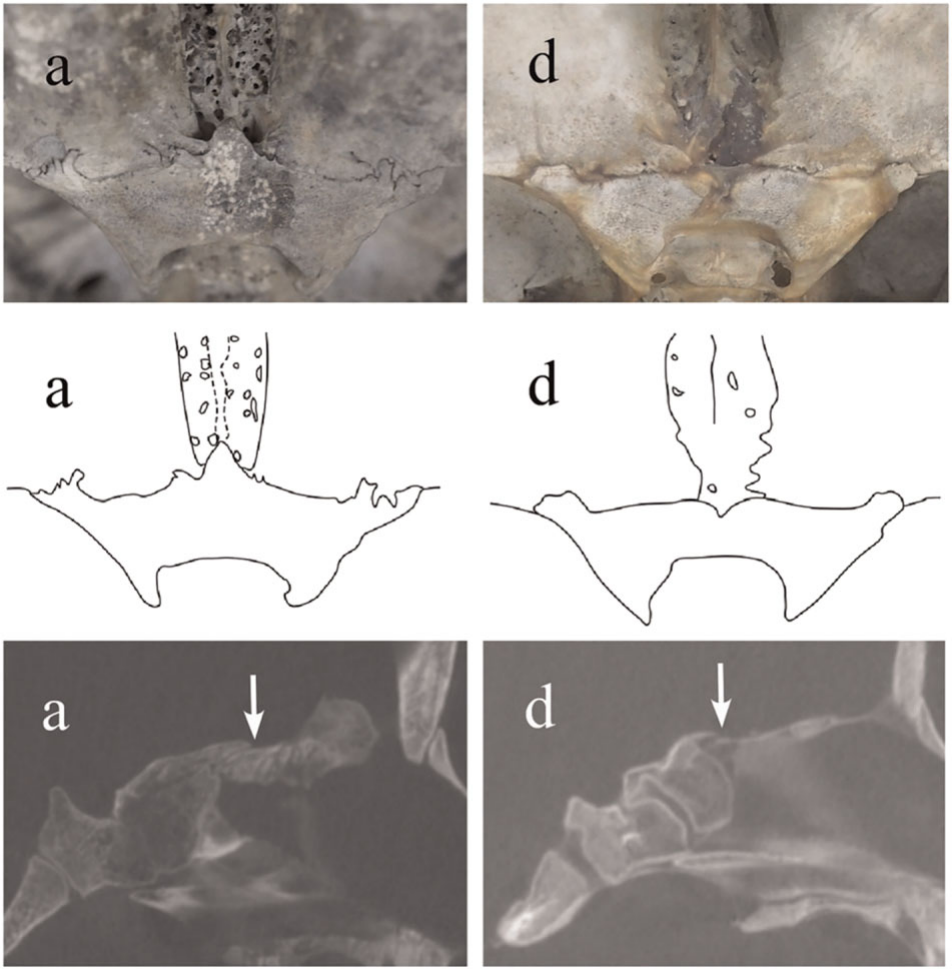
Morphological variations in the anterior cranial fossa in skulls from individuals in early childhood (4–6 months to 2 years old). Upper row shows endocranial photographs, middle row shows traced images of photographs, and lower row shows micro‐CT in the median sagittal plane. (a) Triangular protrusion of the sphenoid body; (d) anterior border of the sphenoid body is straight and/or slightly concave posteriorly. The boundary between ethmoid and sphenoid bones is clearly evident in all individuals on micro‐CT (arrows). CT: computed tomography
